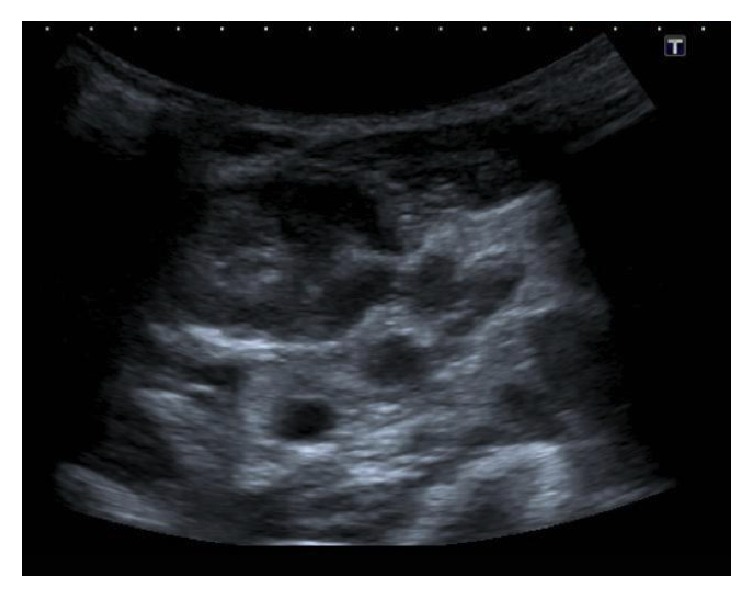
Ultrasound scan showing an enlarged left cervical lymph node with heterogeneous echogenicity.
